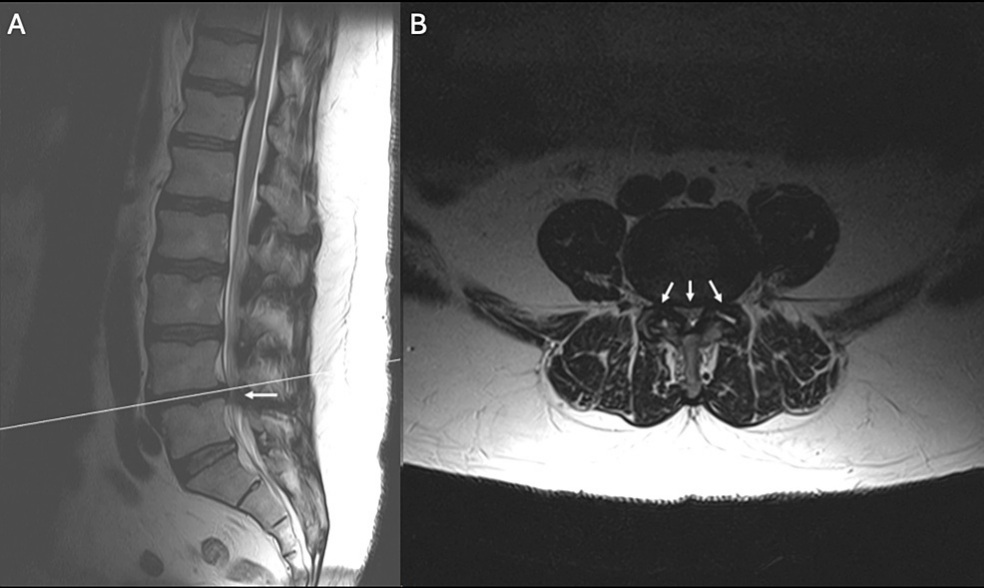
Pre-operative MRI scans of the lumbar spine in sagittal (A) and axial (B) planes demonstrating grade one spondylolisthesis at L4-L5 with notable ligamentum hypertrophy and severe central and arrows marking bilateral neuroforaminal stenosis, as indicated by arrows.
